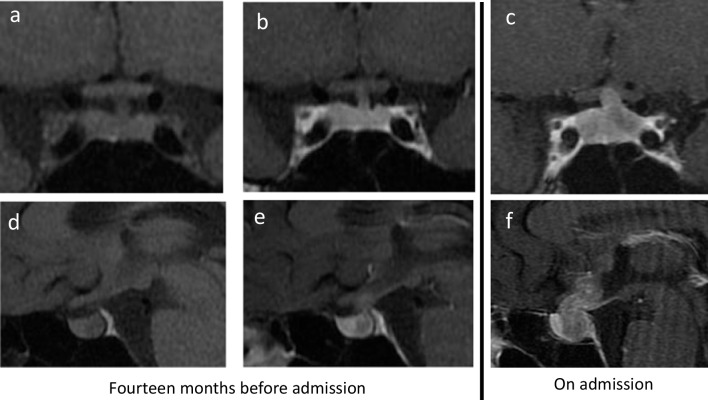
MRI images of pituitary lesion in patient 2. Coronal (a) and sagittal (d) plain T1-weighted images 14 months before admission to our hospital. Coronal (b) and sagittal (e) gadolinium-enhanced T1-weighted images 14 months before admission to our hospital. Coronal (c) and sagittal (f) gadolinium-enhanced T1-weighted images at the time of admission to our hospital. MRI, magnetic resonance imaging
